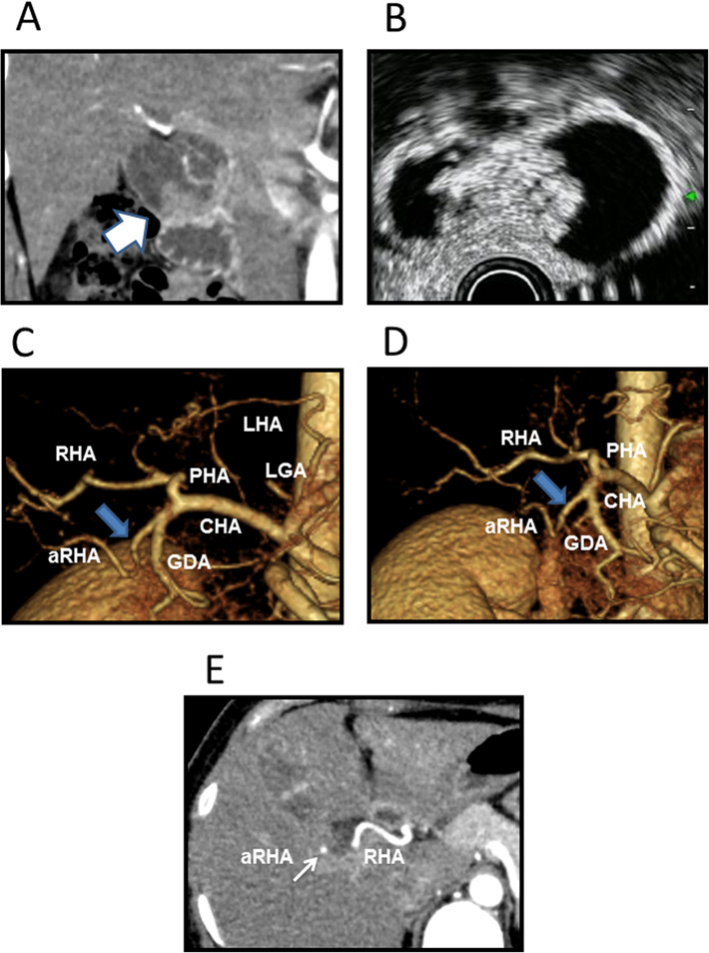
Preoperative findings. A contrast-enhanced CT (a) and ultrasonography (b) revealed a papillary hypervascular tumor, 25 × 21 mm, in the gallbladder. The 3D-CT angiography (c, d) indicated that the aRHA (*arrow*) branched from the GDA, whereas the cholecystic artery could not be detected. The aRHA (*arrow*) fed into the anterior segment of the liver (e). *CHA* common hepatic artery, *GDA* gastroduodenal artery, *PHA* popper hepatic artery, *aRHA* accessory right hepatic artery, *LGA* left gastric artery, *LHA* left hepatic artery
